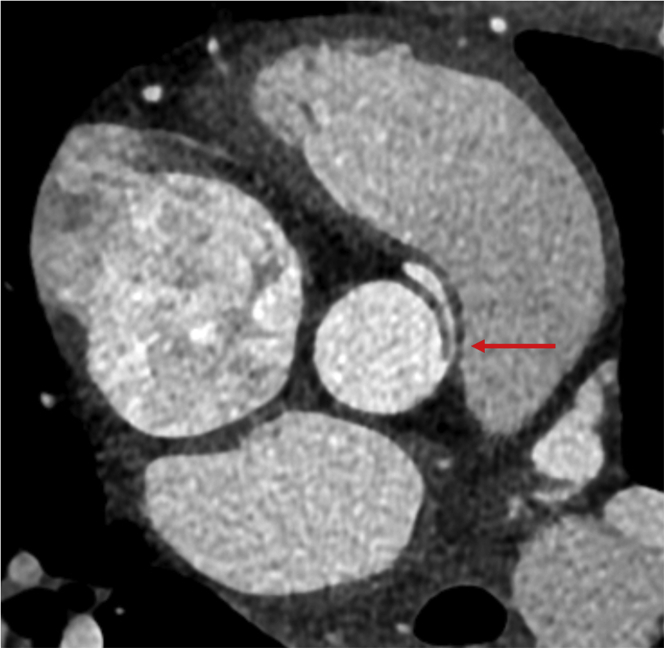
Computed Tomography Coronary Angiography Axial Multiplanar Reformat of the Right Coronary Artery Computed tomography coronary angiography demonstrates the slitlike, proximal intramural course of the right coronary artery, with an acute take-off (arrow)
